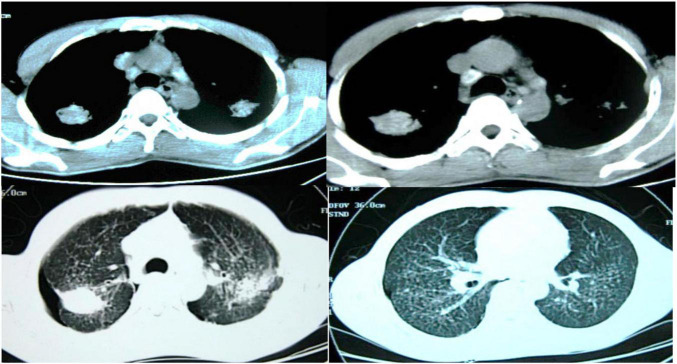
CT images of a 38-year-old male patient.
